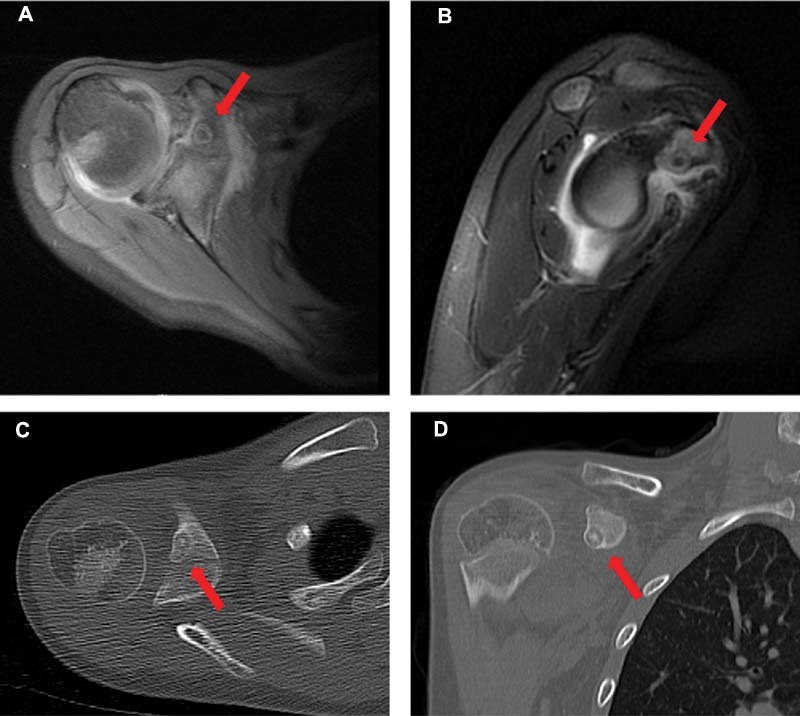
T2-weighted magnetic resonance imaging (MRI) and computed tomography (CT) images of the right shoulder demonstrating an osteoid osteoma. Red arrows point to location of lesion. (
